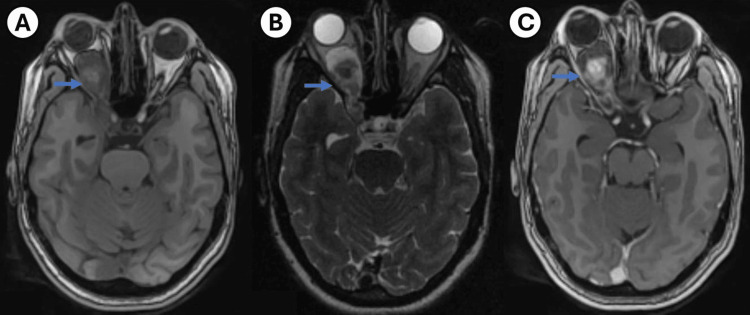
Brain MRI images Panels A and B show significant proptosis of the OD, with noticeable pupil deviation on the same side. The signal intensity within the intraconal space is highlighted, revealing its connection to the intracranial area through the optic foramen (blue arrows). Panel C displays heterogeneous contrast uptake in the inner portion of the intraconal lesion (blue arrow).
